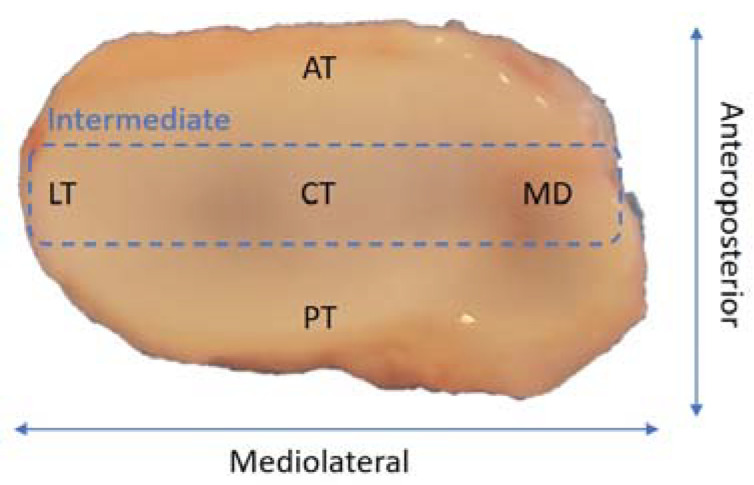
Different regions of the temporomandibular disc: anterior (AT), posterior (PT), lateral (LT), central (CT) and medial (MD).
